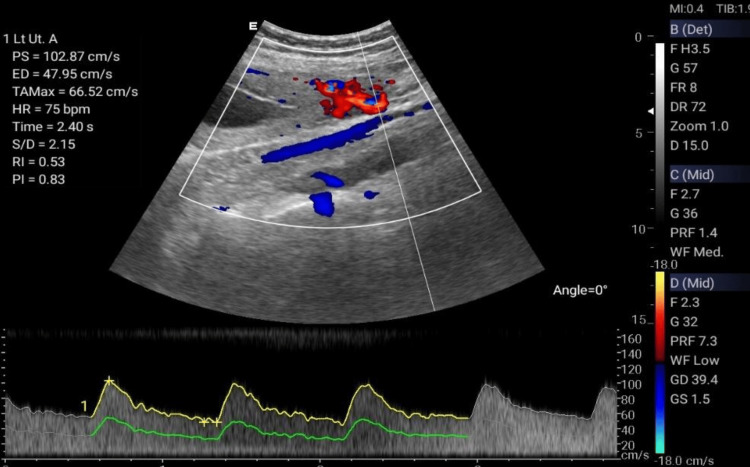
Left uterine artery Doppler.
